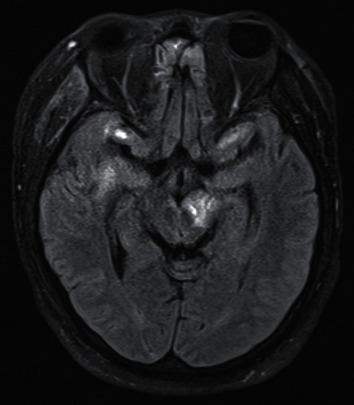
Axial FLAIR and sagittal T1WI MRI showing a significant injury to the left midbrain and multiple intraparenchymal contusions in the frontal, temporal, and parietal lobes.
